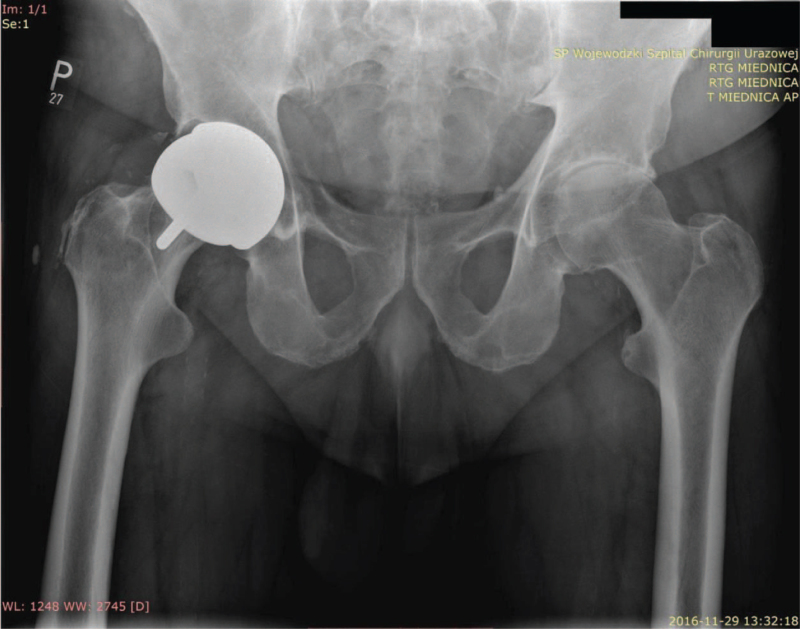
X-ray examination of the pelvis with hip joints in the AP projection. Visible lysis changes around the acetabular component of the right hip joint at the time of admission to the Orthopaedic Trauma Emergency Room, degenerative changes in the left hip joint.
